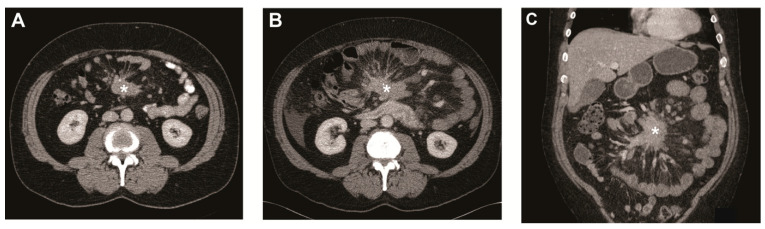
Metastatic mesenteric mass and surrounding fibrosis over time. (A) Transverse image of CT scan at baseline showing mesenteric mass (asterisk) with radiating strands of fibrotic tissue. Transverse (B) and coronal image (C) of CT scan after 5 years showing the mesenteric mass (asterisk) growth of >20% on the short axis.
